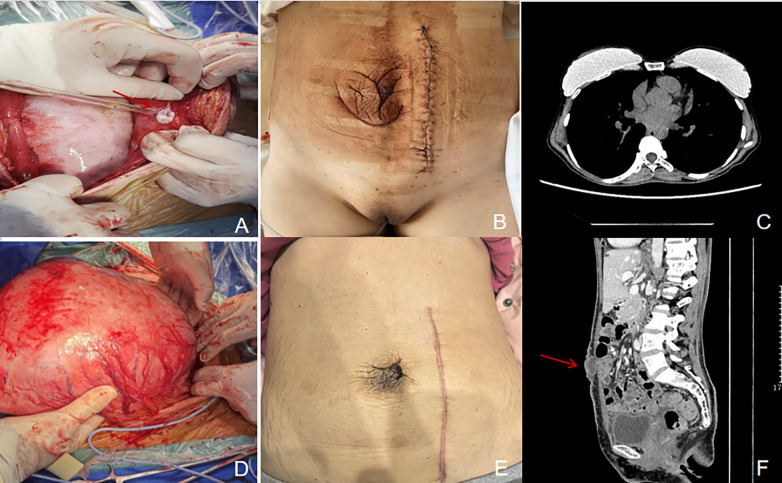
Postoperative follow-up imaging and intraoperative findings. (A) Intraoperative view showing the large fascial defect on the abdominal wall corresponding to the umbilical hernia (arrow). (B) Clinical photograph one week postoperatively, demonstrating significant retraction of the umbilicus and complete resolution of the posterior vaginal wall prolapse. (C) Axial chest CT image at one-month follow-up, revealing complete resolution of bilateral pleural effusions. (D) Intraoperative photograph of the giant uterine leiomyoma being delivered out of the abdominal cavity. (E) Clinical photograph at ten-month follow-up, showing near-normal restoration of umbilical anatomy without surgical repair. (F) Sagittal reconstruction of abdominal CT at three-month follow-up, showing a residual fascial defect at the umbilicus (red arrow), measuring approximately 36 × 11 mm.
